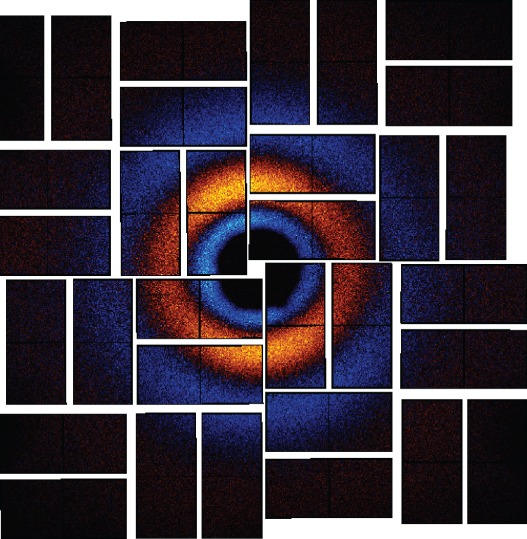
Difference image at the X-ray detector (CSPAD) for a delay of approximately 100 fs after pumping with 800 nm light.
